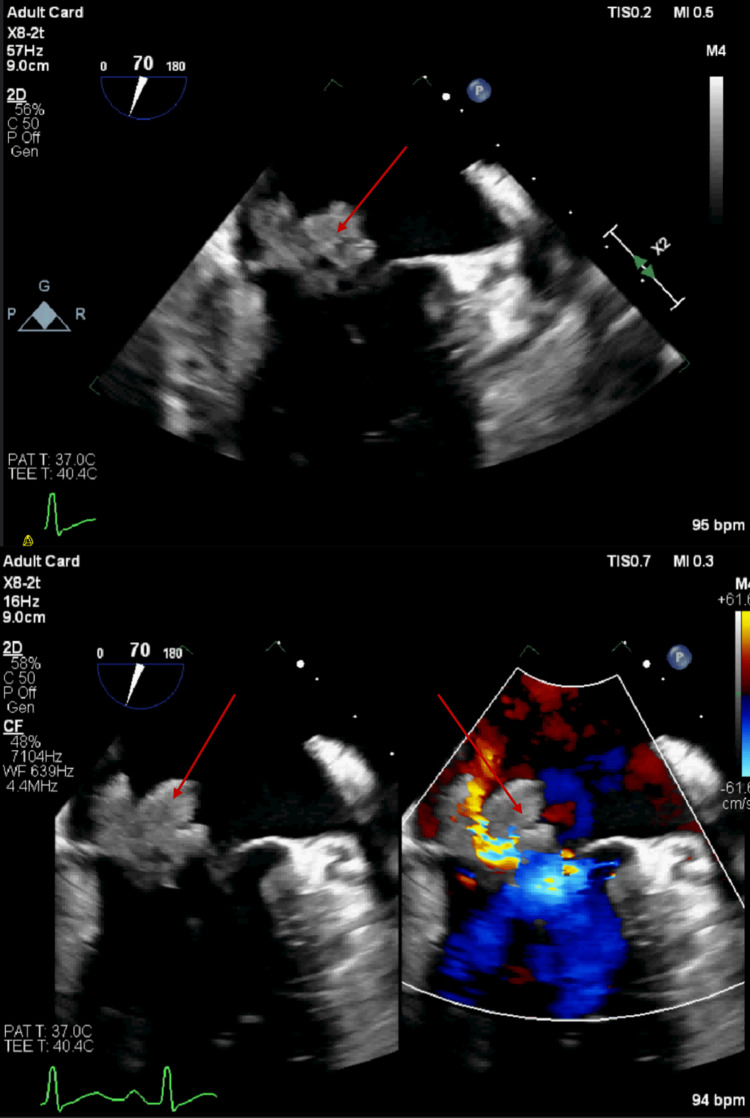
A transesophageal echocardiogram showing large vegetations on both the anterior and posterior leaflets Red arrows indicate valvular vegetation
